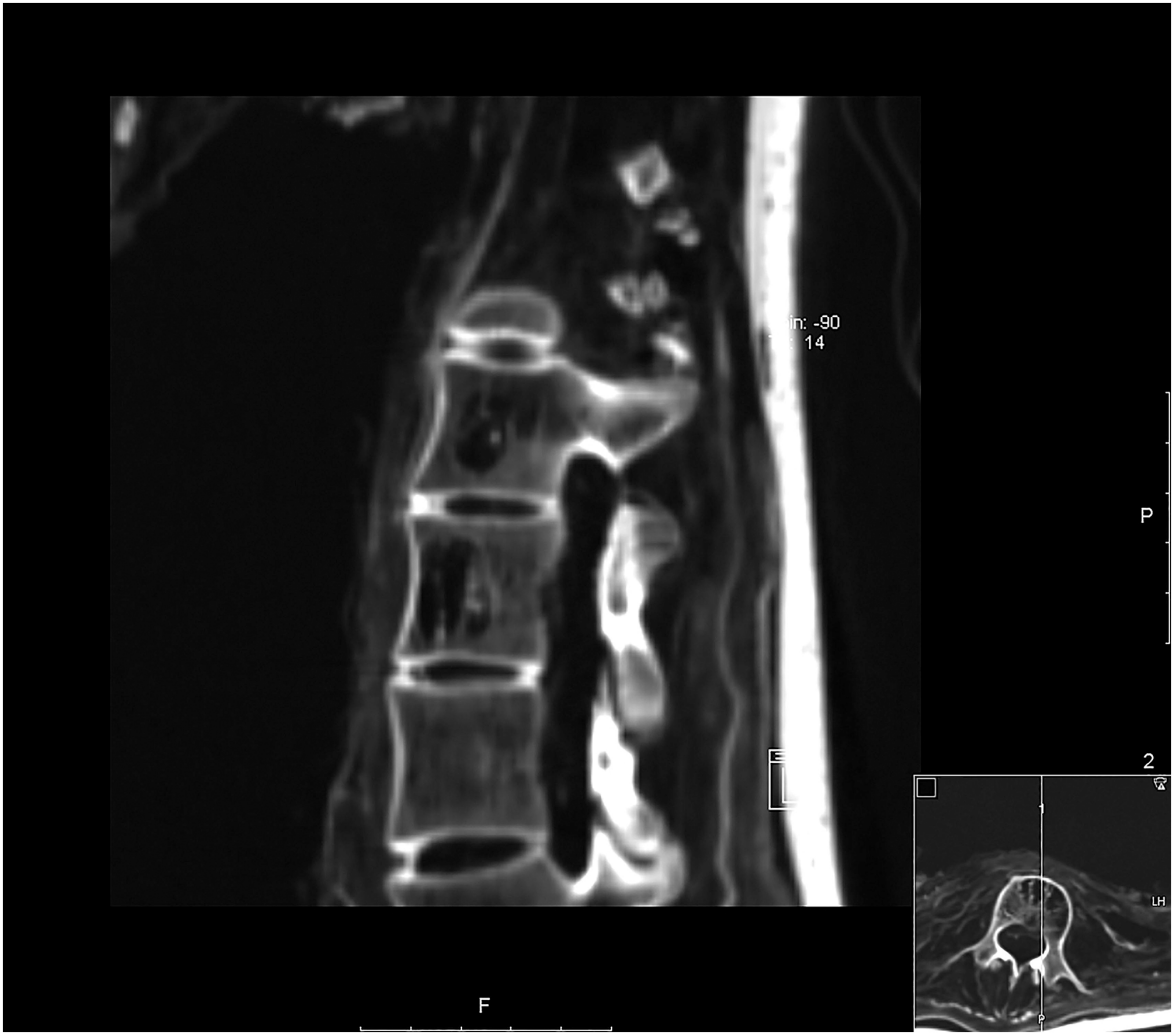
Sagittal multi-planar reconstruction of a CT data set showing the lumbar spine of individual C. In the sagittal view, the lumbar vertebrae 1 and 2 show sharply confined lesions of honeycomb appearance, also visible in the axial view (small window). The lesions were probably caused by intraosseous vertebral haemangiomas.
